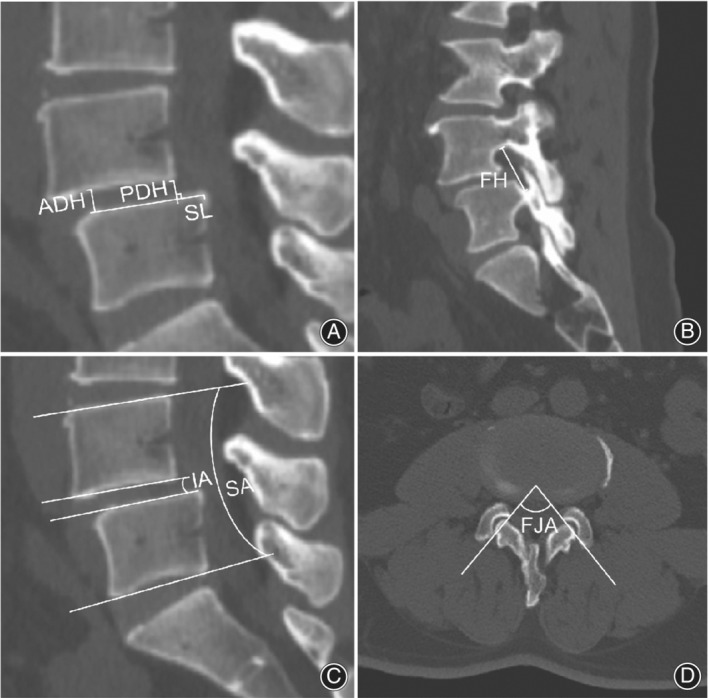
Measurement of radiographic parameters. (A) Anterior disc height (ADH), posterior disc height (PDH), DH = (ADH + PDH)/2, slippage length (SL). (B) Foramen height (FH). (C) Segmental angle (SA), intervertebral angle (IA). (D) Facet joint angle (FJA)
